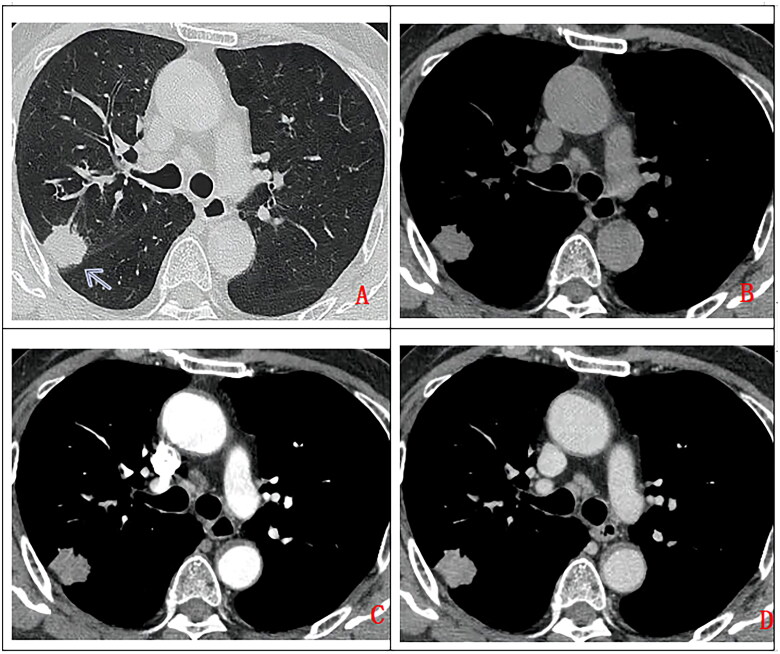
A 68-year-old man, with no clinical symptom had chest CT scan without contrast (A. lung window, B. mediastinum window) and with contrast (A. artery phase, B. Delay phase). a solitary subpleural pulmonary nodule in right upper lobe (as indicated by arrow), with lobulated margin, uniform density, moderate enhancement on contrast enhancement CT. Pathologically confirmed high-grade mucoepidermoid carcinoma after surgical operation.
